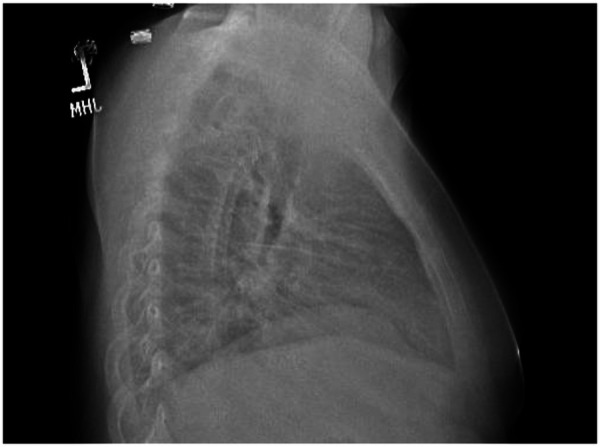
Chest xray lateral view demonstrating bilateral pleural effusions and trace pericardial effusion at presentation.
